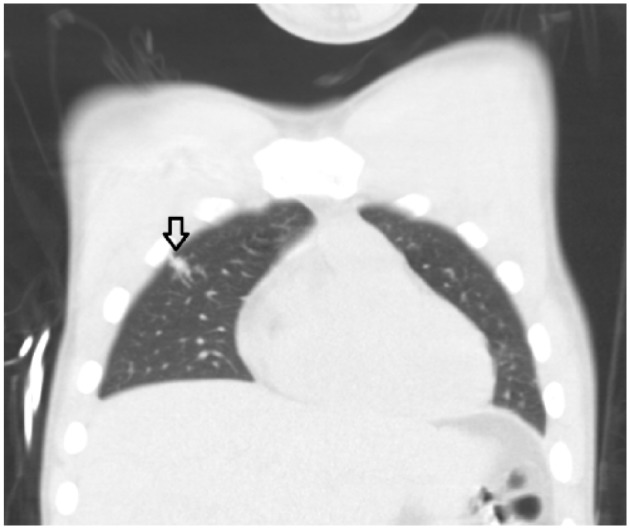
Coronal section of CT chest shows a serpiginous focus within the right upper lobe.
